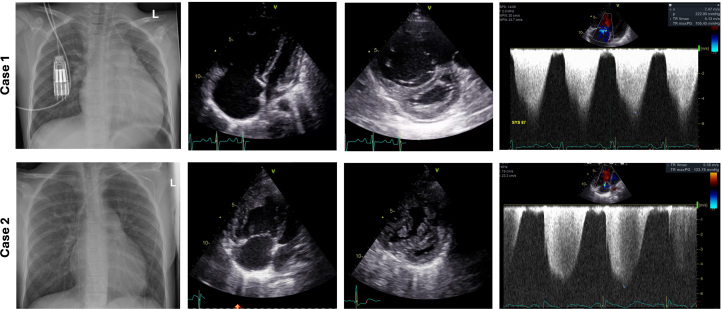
Imaging Findings in Patients With Pulmonary Arterial Hypertension Radiographic and echocardiographic imaging for (Top Row) case 1 and (Bottom Row) case 2. Both patients showed cardiomegaly and prominent PA segment, RA and RV dilatation, septal flattening, and suprasystemic RV pressure. PA = pulmonary artery; RA = right atrial; RV = right ventricular.
